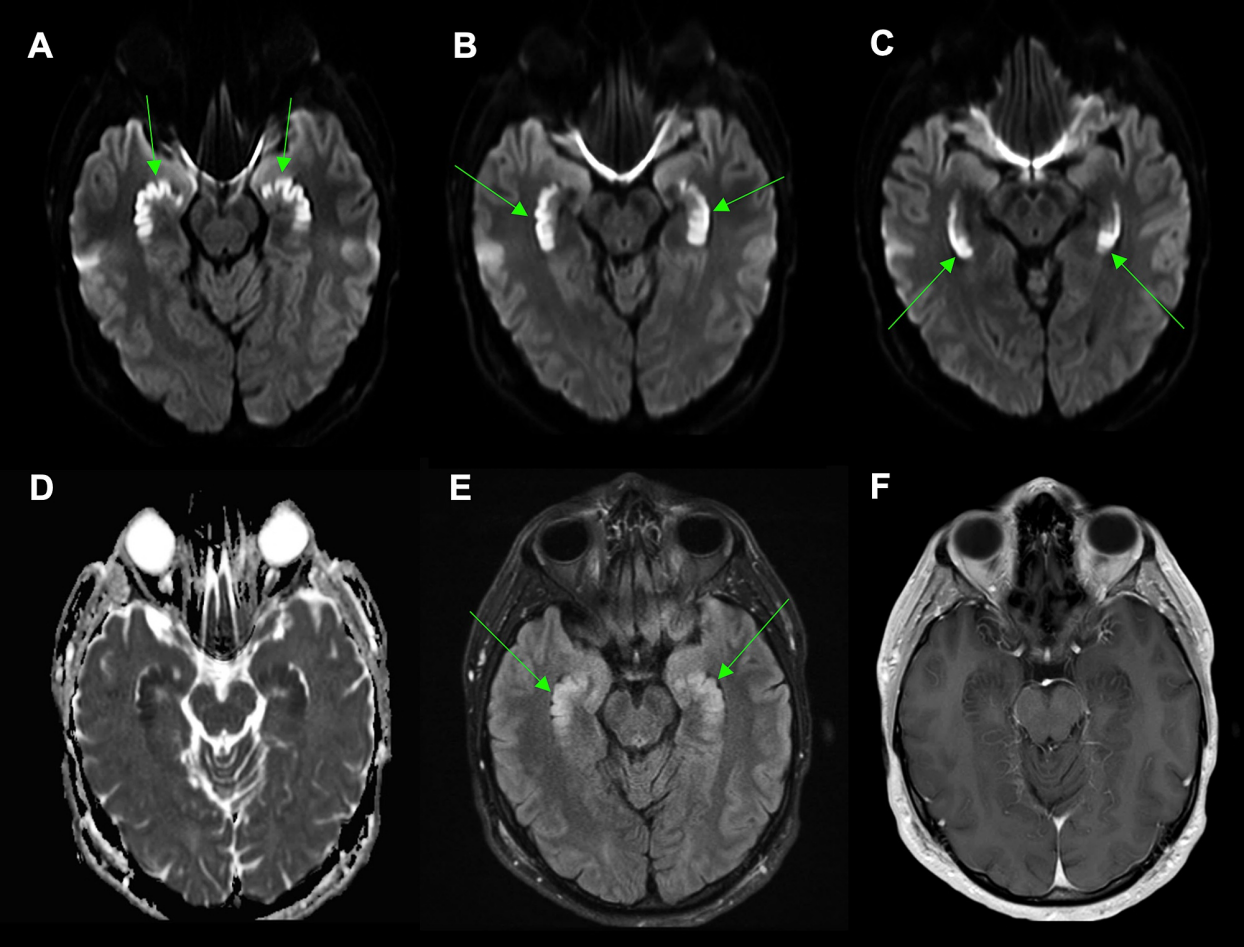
Magnetic resonance imaging of the brain. Diffusion-weighted imaging (DWI) demonstrates bilateral, symmetric hyperintensity (arrows) in the (A) heads, (B) bodies, and (C) tails of the hippocampi. (D) The apparent diffusion coefficient (ADC) correlate map shows a corresponding low signal, consistent with diffusion restriction. (E) Abnormal T2-fluid-attenuated inversion recovery (FLAIR) hyperintensity is present in the hippocampi (arrows). (F) Axial T1-weighted imaging shows no abnormal contrast enhancement.
